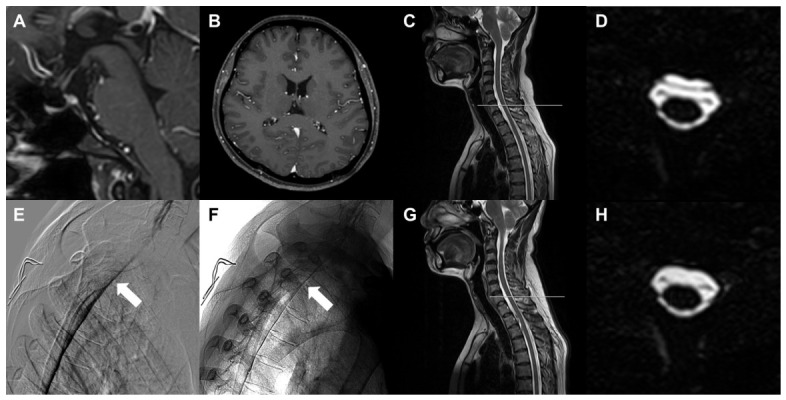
A case of a 55-year-old female with Type 1 leak. (A,B) Preoperative brain MRI demonstrating normal intracranial appearances with a Bern score of 0, despite the patient’s persistent symptoms. (C,D) Preoperative MR myelography showing an organized ventral spinal longitudinal extradural collection (SLEC) extending from C5 to T4. (E,F) Digital subtraction myelography images localizing the rapid ventral CSF leakage at the T2–3 level (white arrows). (G,H) After two months of surgery, an MR myelography confirming complete resolution of the SLEC.
